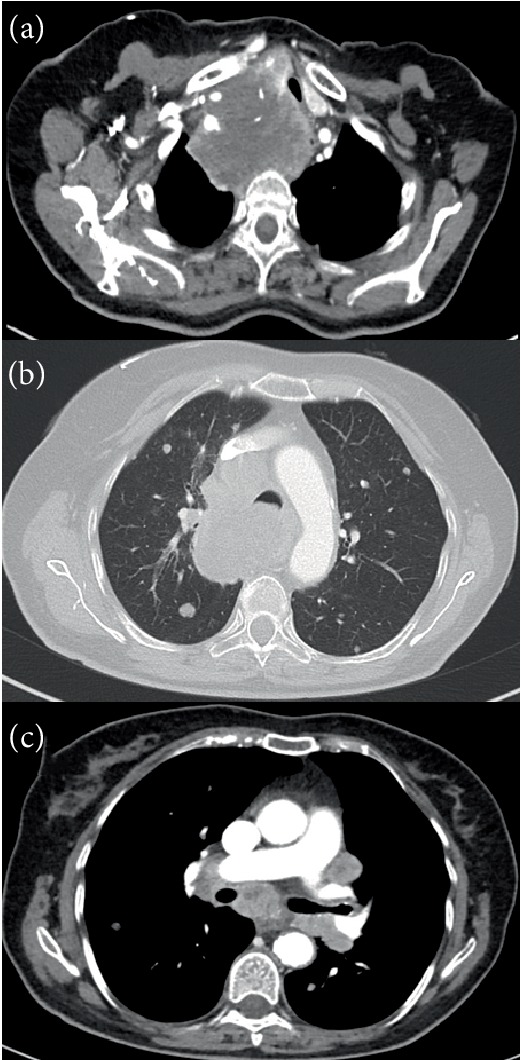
Baseline chest CT showing a large mass originating from the right thyroid lobe (a), with lung metastases (b), and hilar and mediastinal lymph nodes (c). (a), (c) images in mediastinal window. (b) image in lung window.
